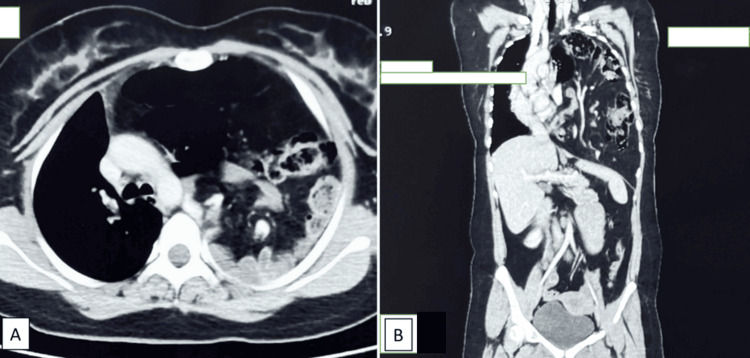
Preoperative contrast-enhanced CT scan: (A) coronal and (B) axial sections of the scan showing herniation of the abdominal contents into the left thoracic cavity
